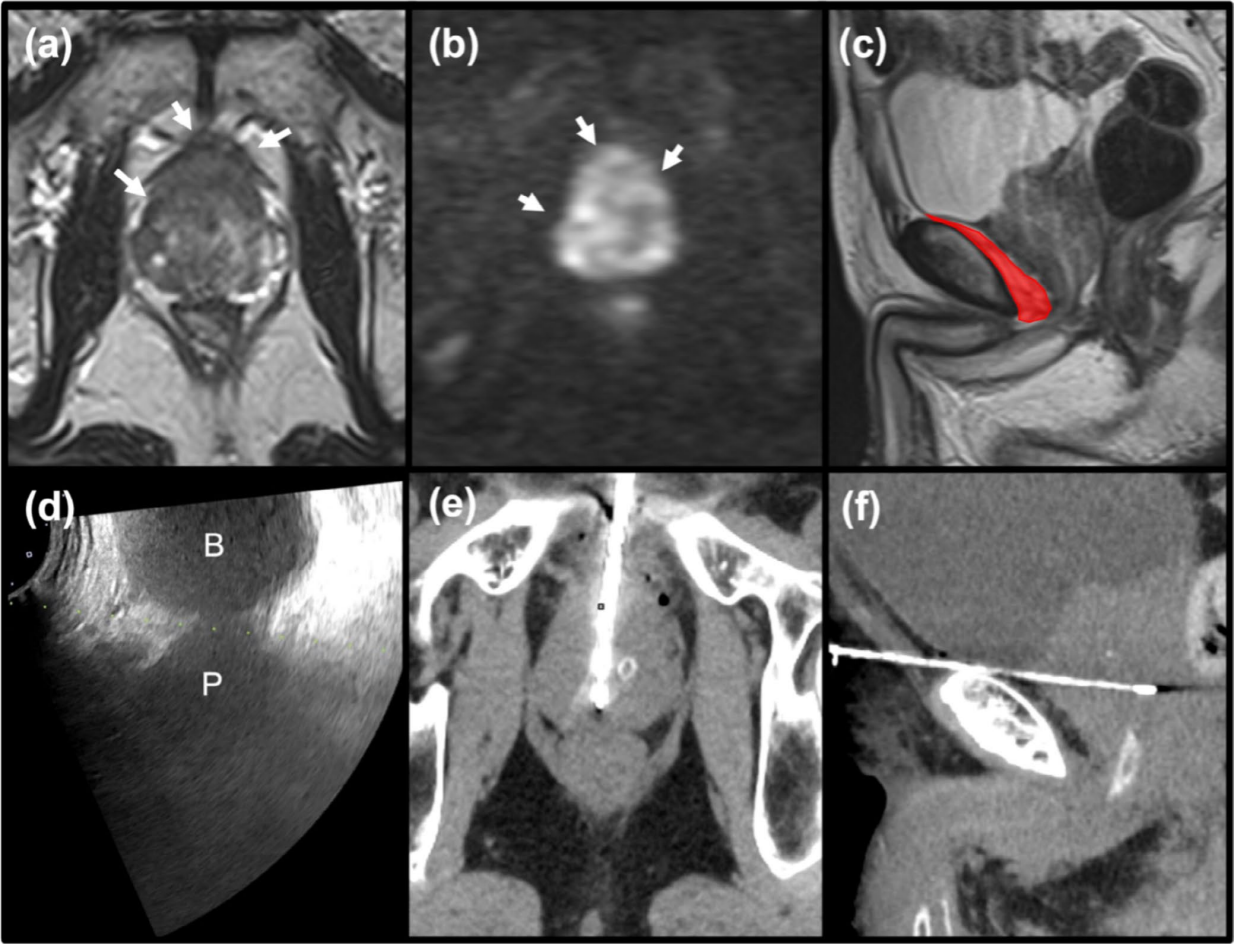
(a, b) Axial T2‐weighted image shows homogeneous hypointensity and diffusion weighted image shows hyperintensity in the anterior zone of the prostate, suggesting prostate cancer (arrows). (c) Sagittal T2‐weighted image shows the retropubic space (red). (d) Transabdominal US showing the bladder (B) and prostate (P). The needle is advanced along the virtual puncture line (dashed line). (e, f) CT reconstructed in the oblique axial and oblique sagittal planes parallel to the needle when the biopsy needle reached the prostate shows that the biopsy needle reached the right side of the prostate, avoiding the urethral catheter.
